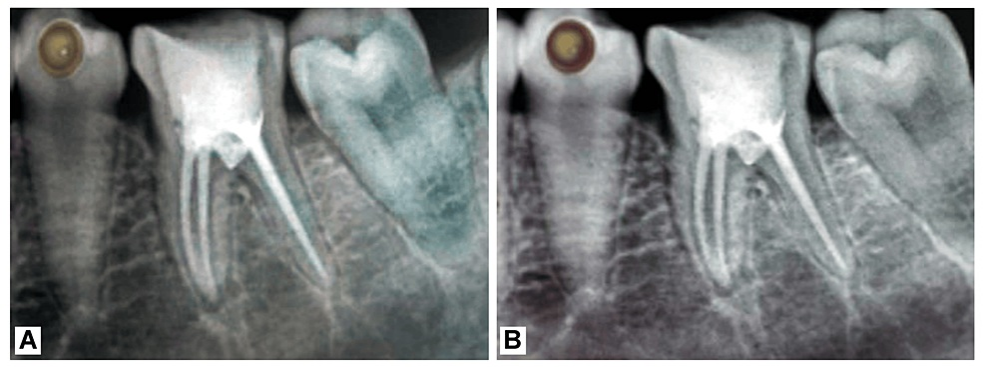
Post-operative radiographs: (A) immediate post-operative radiograph of 46; (B) six-month follow-up post-operative radiograph of 46 Courtesy: Shreeya Panchal
